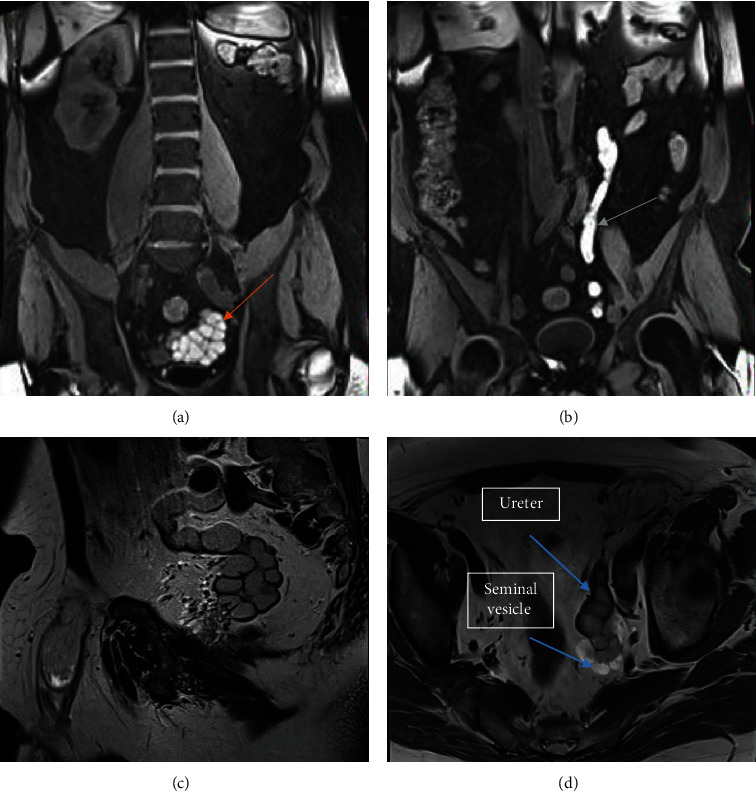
(a) MRI T1 coronal section showed left renal agenesis and dilatation of the left seminal vesicle (red arrow) that shows high signal content, suggesting hemorrhagic content or a high proteinaceous component; (b) tubular structure (green arrow) in the left lumbar and pelvic region that seemed to correspond to the dilated ureter (c). MRI T2 sagittal section reveals the ureter empties ectopically into the left seminal vesicle, which is markedly enlarged and multicystic and (d) shows in the axial the contrast enhancement difference between the ureter and seminal vesicle.
